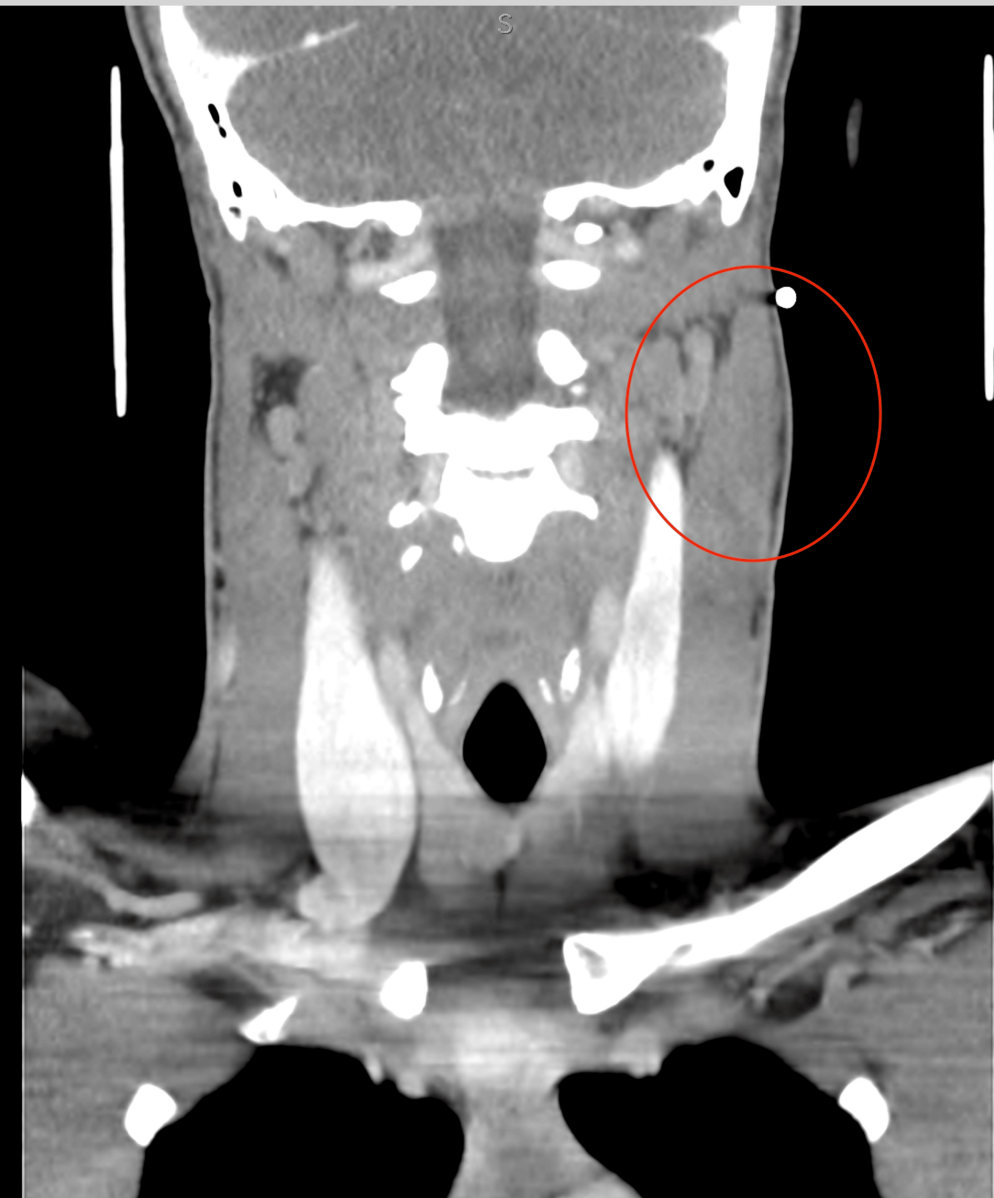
Contrast-enhanced CT showing a multiloculated cystic lesion (circled in red) between the left parotid tail and the sternocleidomastoid muscle.
